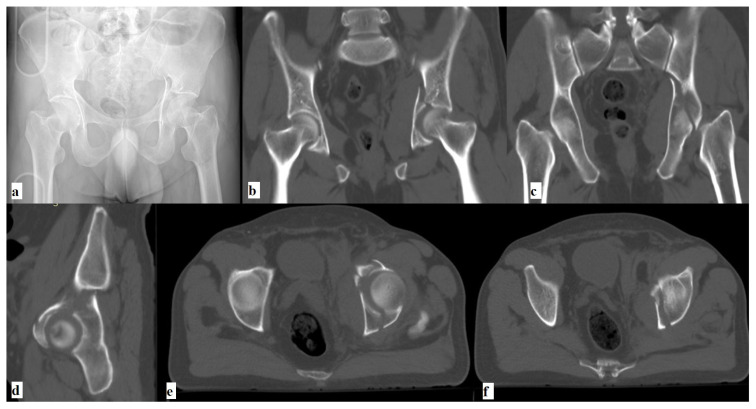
(a) Preoperative radiographs and (b,c,d,e,f) CT images of a 51 year old male patient who suffered from a traffic accident showing left anterior column/posterior hemitransverse acetabular fracture with acetabulum posterior wall fracture.
